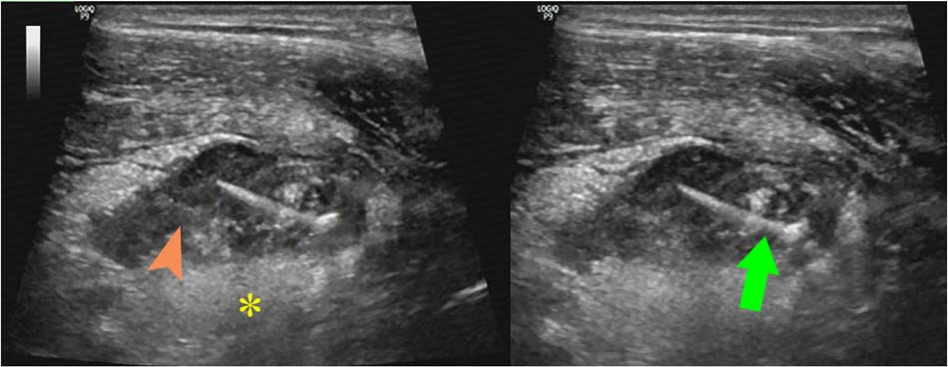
Ultrasound images showed a large pus-filled (arrowhead) appendix with a thin internal echogenic structure (arrow) extending outward from the muscle layer with surrounding fat infiltration (asterisk).
